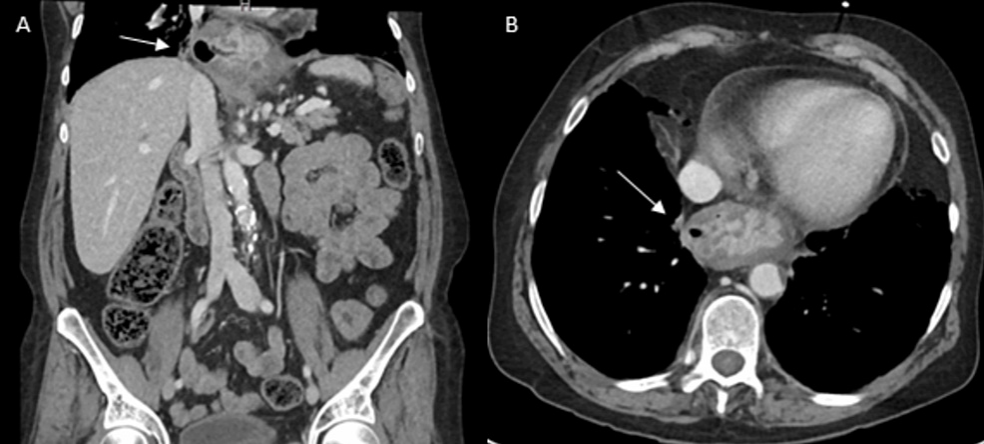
CT Abdomen and Pelvis The image on the left (A) is a coronal CT with intravenous contrast and the image on the right (B) is an axial view of the same CT study. In both images, the arrows point to the stomach that has herniated into the thoracic cavity through the crura of the diaphragm.
